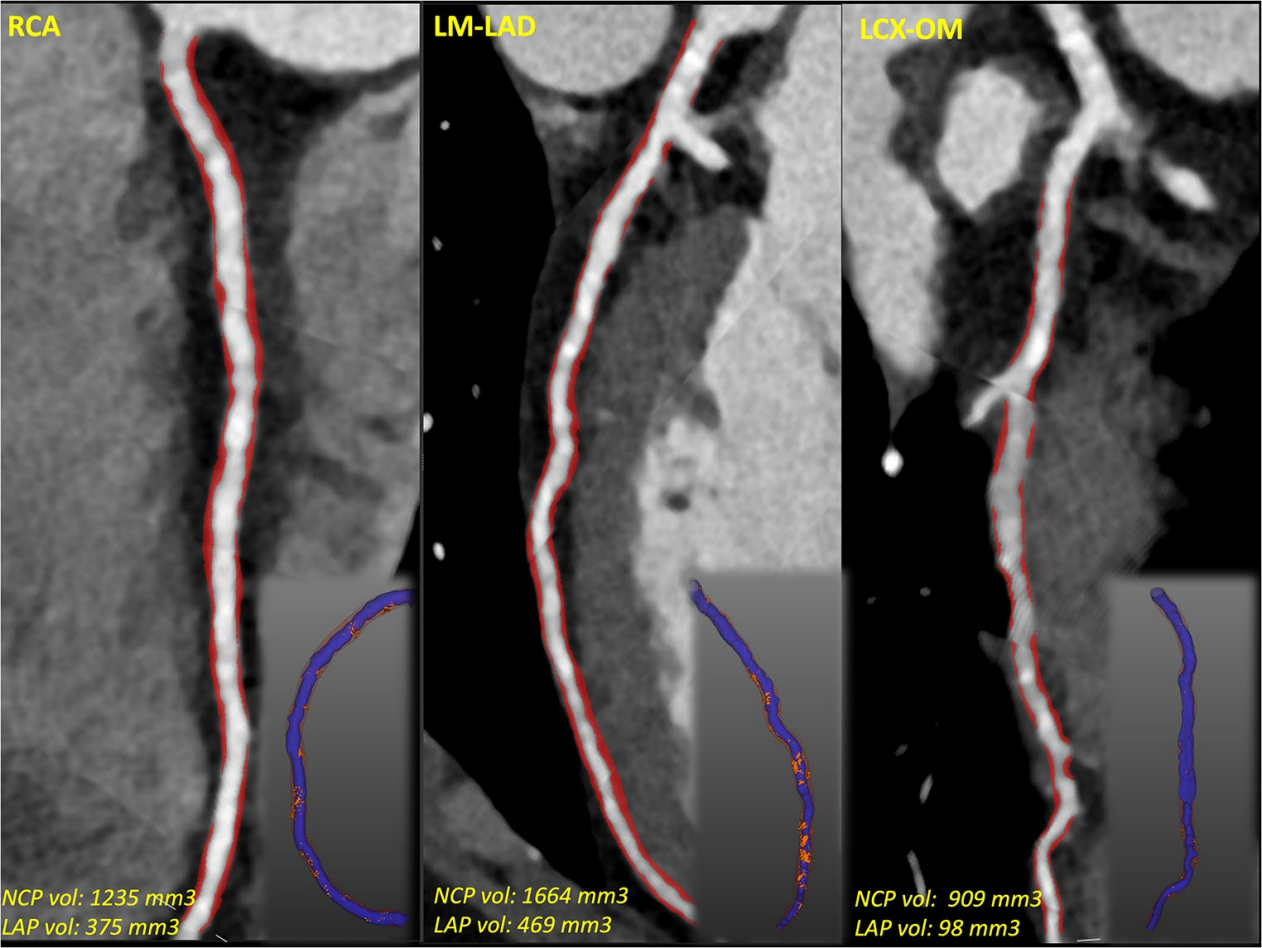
Coronary computed tomography angiography (CCTA) 3 years prior event. Main panels: Curved multiplanar reformations of 3 main epicardial vessels after advanced plaque quantification analysis, showing per-vessel type and volume of plaque. Miniature panels: 3D representation of the corresponding panel vessel depicting lumen in blue, non-calcified plaque (NCP) in red, and low attenuation plaque (LAP) in orange
